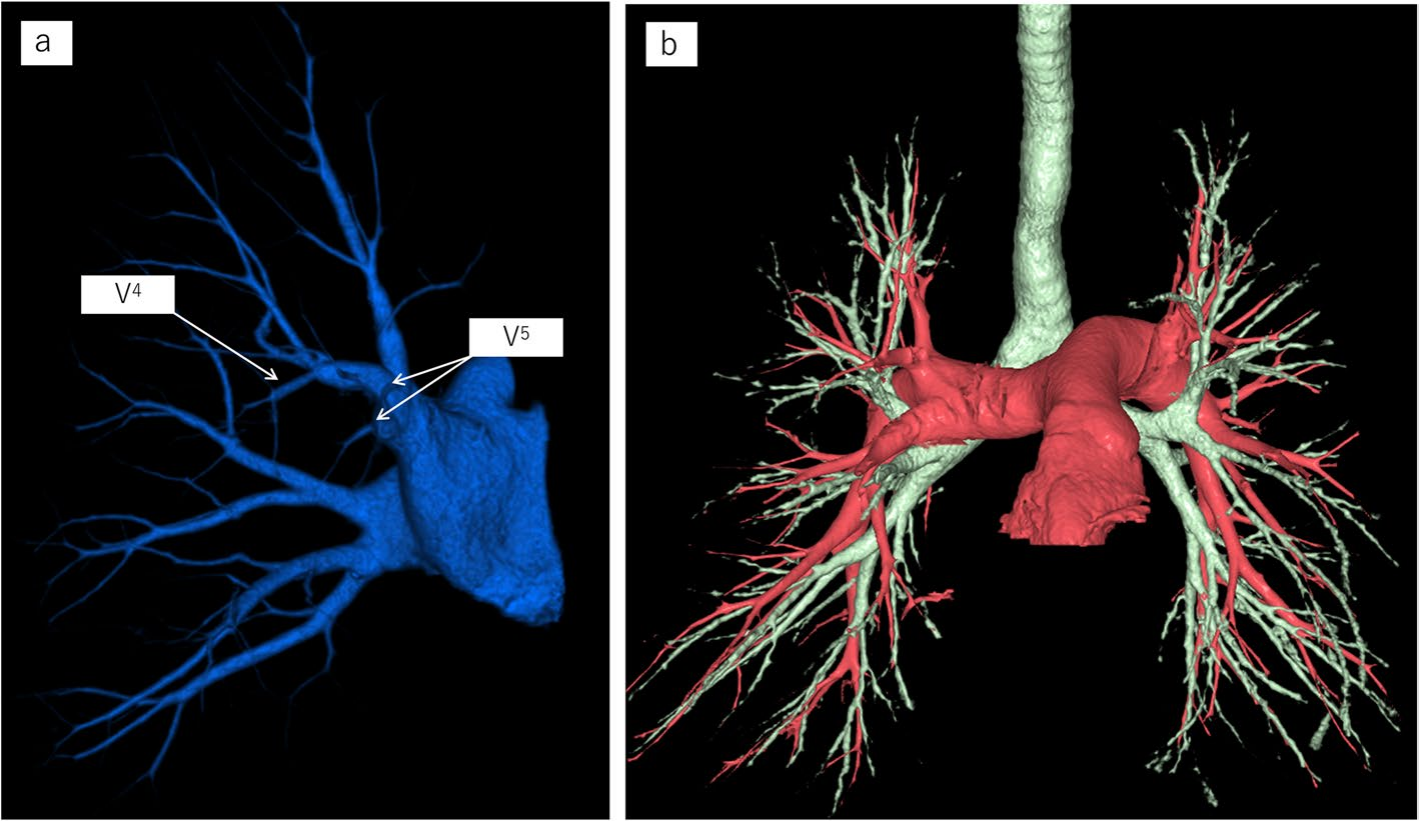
3D-CT revealed that V^4^ was connected to V^2^, and V.^5^ was independently draining into the superior pulmonary vein (a). The right PA is a common trunk with the mediastinal type of the right PA. The common trunk supplies the RML, which is similar to the mediastinal lingular artery of the left PA (b)
